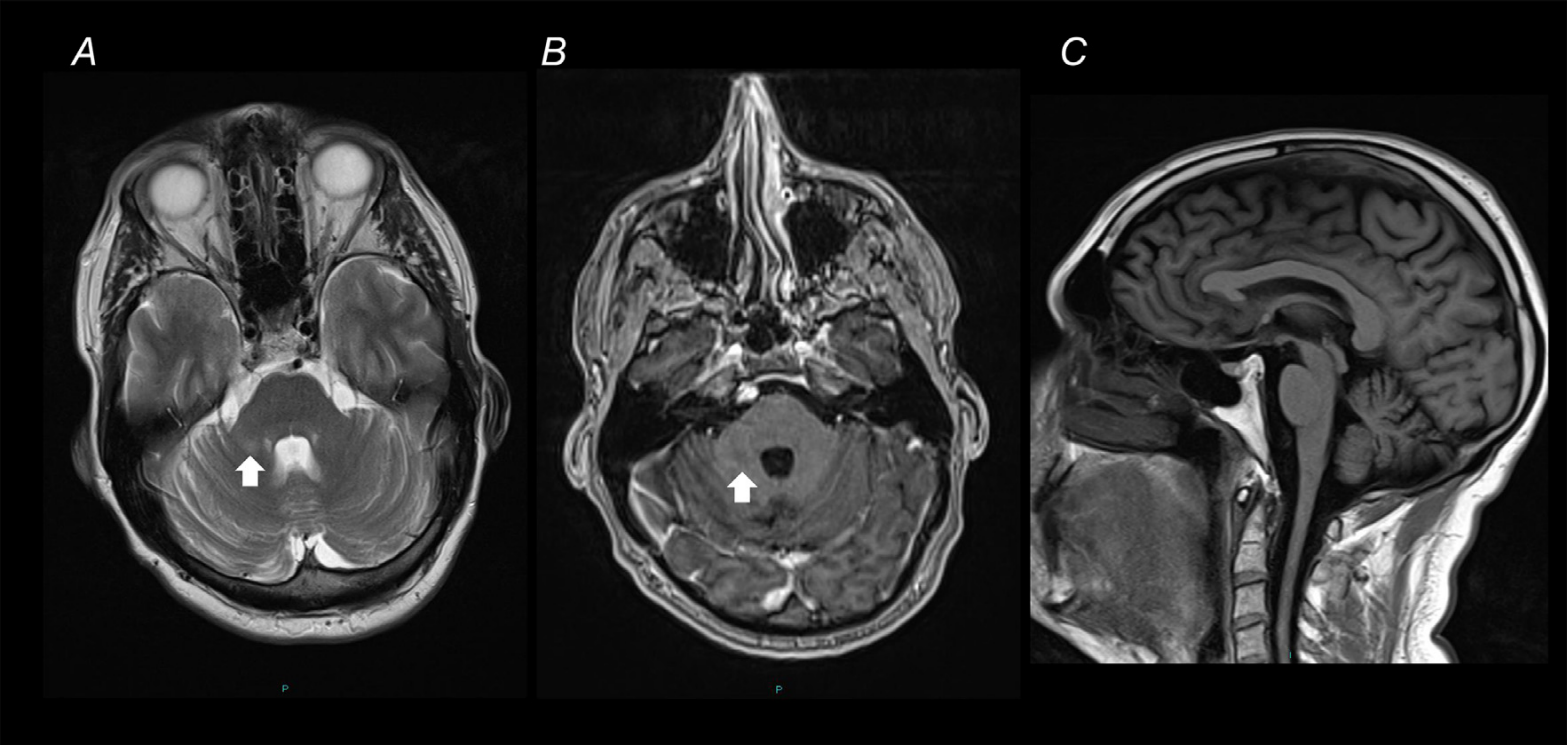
Cranial MRI scans. (A) Axial view T2-weighted sequence showing a hyperintensity in right middle cerebellar peduncle, without evidence of contrast enhancement (arrow) as shown in (B), the T1-weighted sequence. (C) Sagittal view T1 sequence showing mild cerebellar atrophy.
